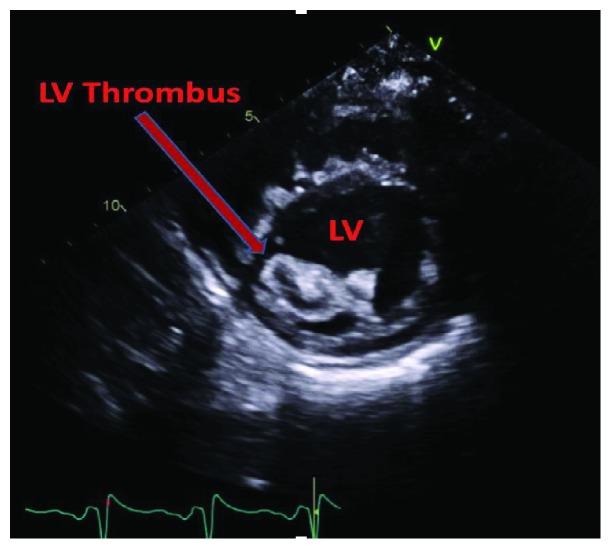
Parasternal short axis view. Mid to apical segment showing LV thrombus. LV: left ventricle.
